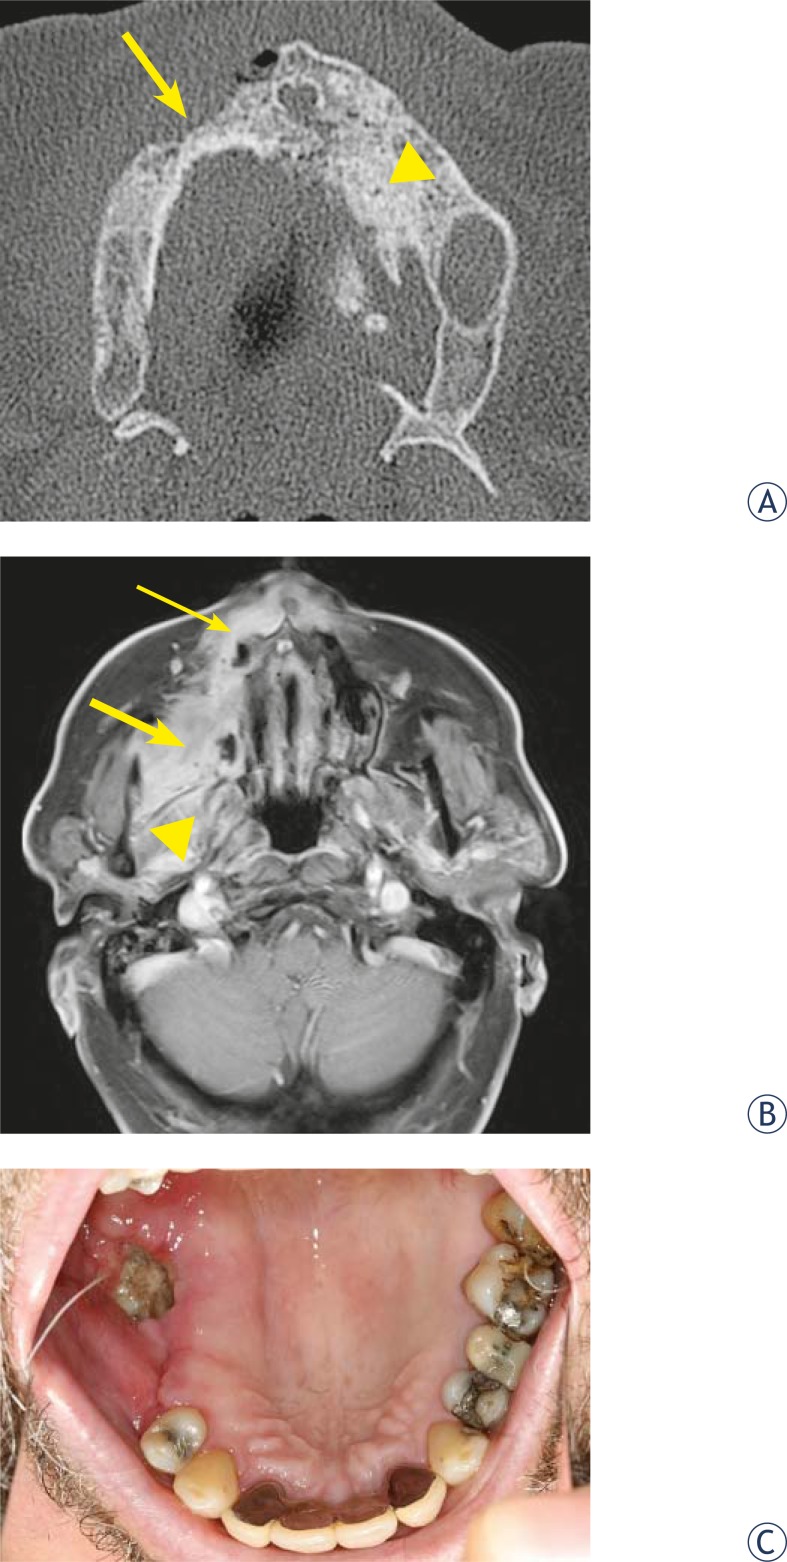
CT and MRI image of a 78-year-old man with multiple myeloma. (A) Axial CT image reveals osteolytic region in the right maxilla (arrow). There is periostal bone reaction, as well as a sclerosis of the maxilla bone marrow on the left side (arrowhead). (B) The corresponding axial T1-weighted, fat-saturated, contrast-enhanced, MRI image demonstrates enhancement in pterygoid and masseter regions (thick arrow). There is also enhancement of necrotic region in right maxilla (thin arrow) and right mandibular ramus with medial cortex resorbtion and periostal reaction (arrowhead). (C) Exposed infected (Actinomyces) bone in the same patient.
